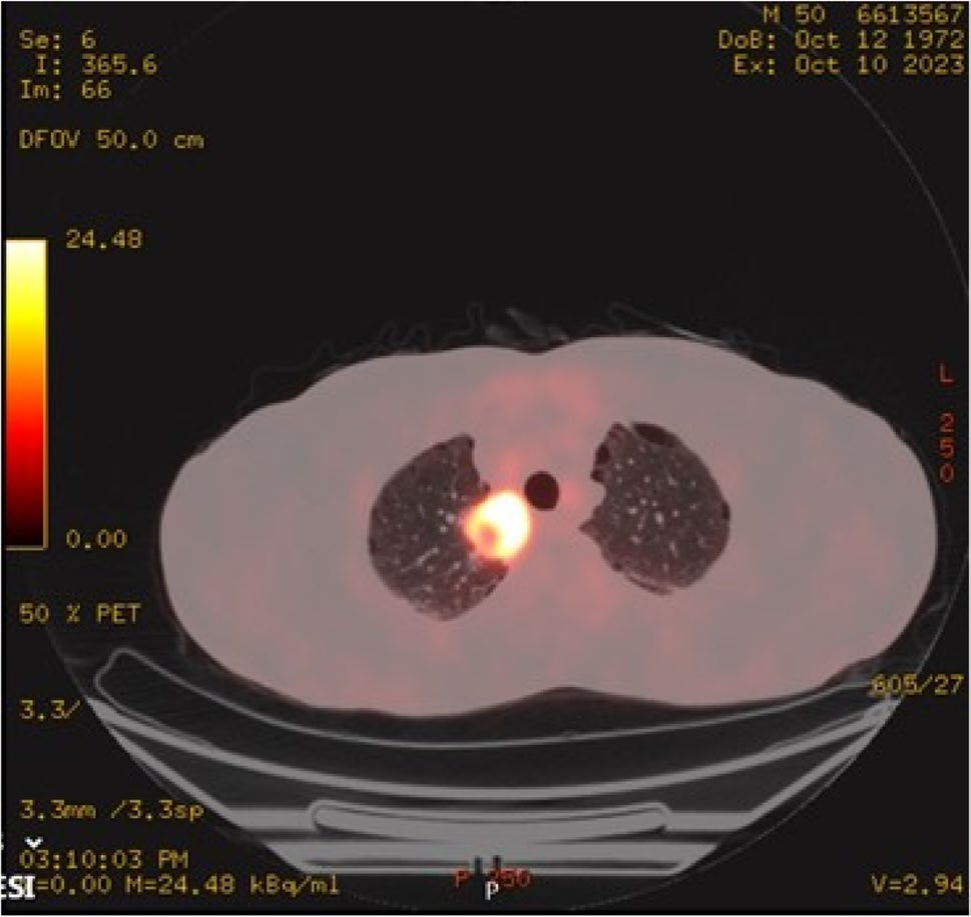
PET-CT imaging: A roughly 3 × 3 cm mass lesion extending from the right upper and lower paratracheal regions into the right upper lobe parenchyma, demonstrating FDG uptake
